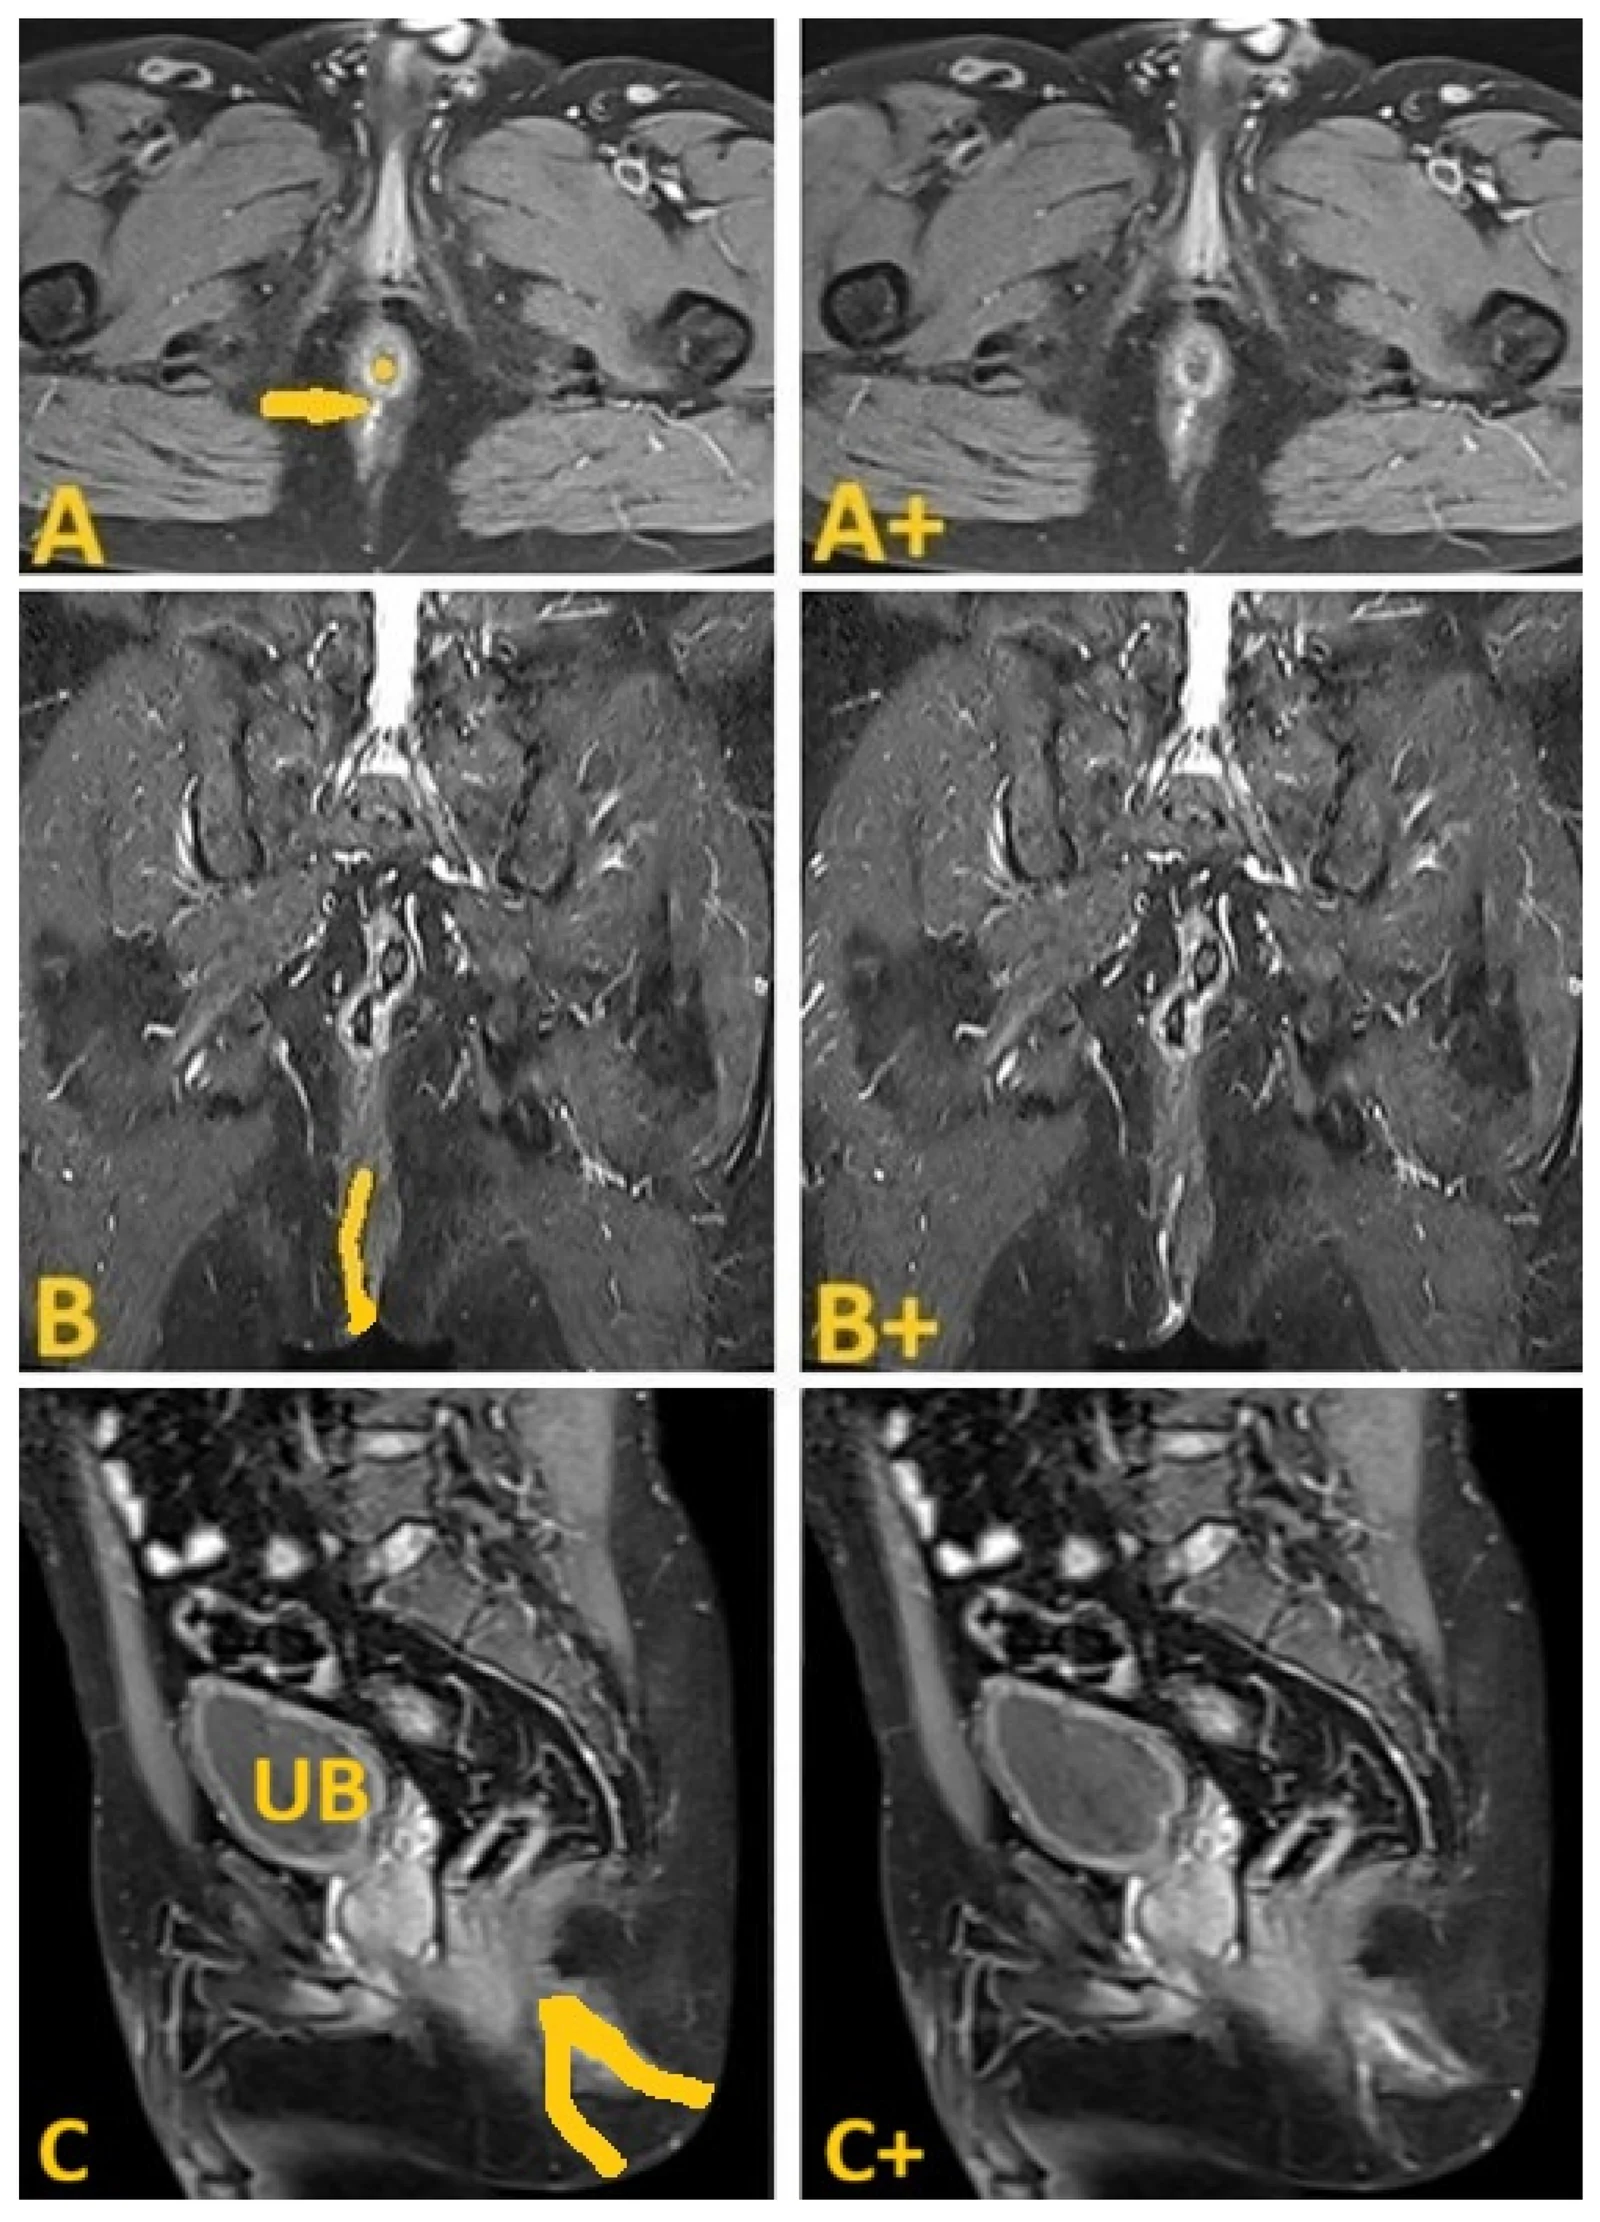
Selected magnetic resonance imaging images. (A) Axial DIXON T1-weighted image showing a perianal fistula arising from the right posterior anal sphincter region at the 7 o’clock position (arrow) around the anal canal (dot) in the lithotomy position, with the internal opening located approximately 35 mm from the anal verge. (A+) Corresponding unannotated image. (B) Coronal STIR T2-weighted image showing an intersphincteric fistulous tract measuring 60 mm in length and 3 mm in width, extending inferiorly in the right posterior quadrant to form two external openings. No abscess formation is identified. (B+) Corresponding unannotated image. (C) Sagittal STIR T2-weighted image showing part of the branching fistulous tract and the two external openings. (C+) Corresponding unannotated image. UB: Urinary bladder.
